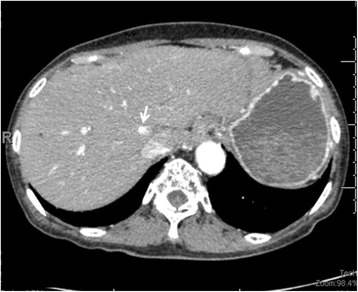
A small HCC (*white arrow*) was detected at the caudate lobe of an 80-year-old female patient with serum high alpha-feto-protein level, which was recognized only by arterial phase of dynamic computed tomography
